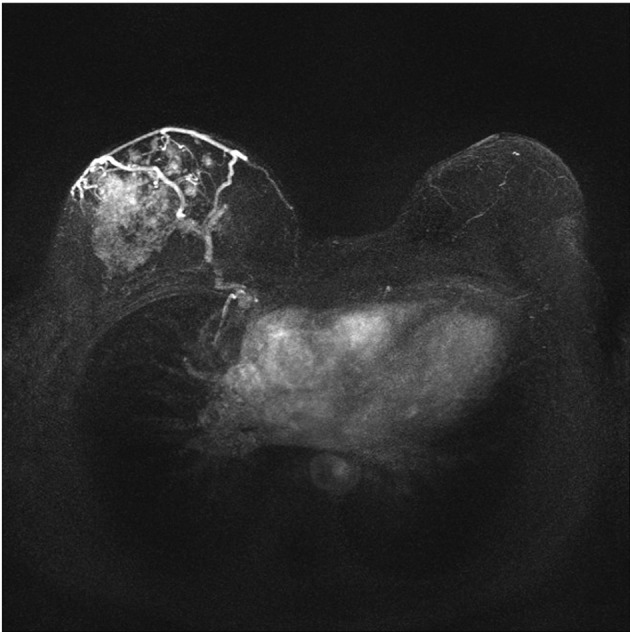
Figure 2. MRI in DCIS of the right breast demonstrating a non-mass-like enhancement using MIP (Maximum intensity projection) in post-processing
